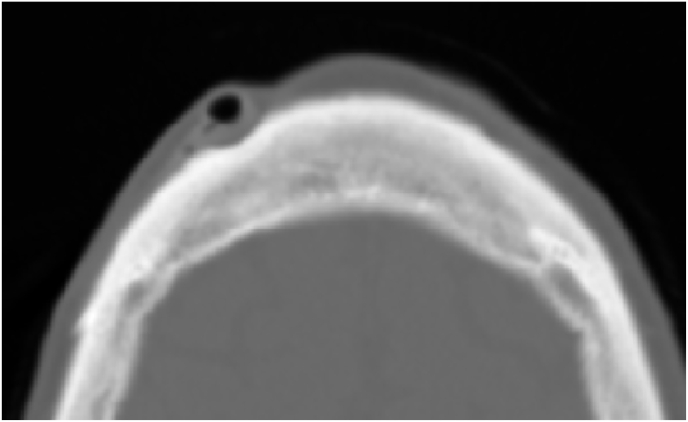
CT SCAN: bublle air in the shunt chamber.
